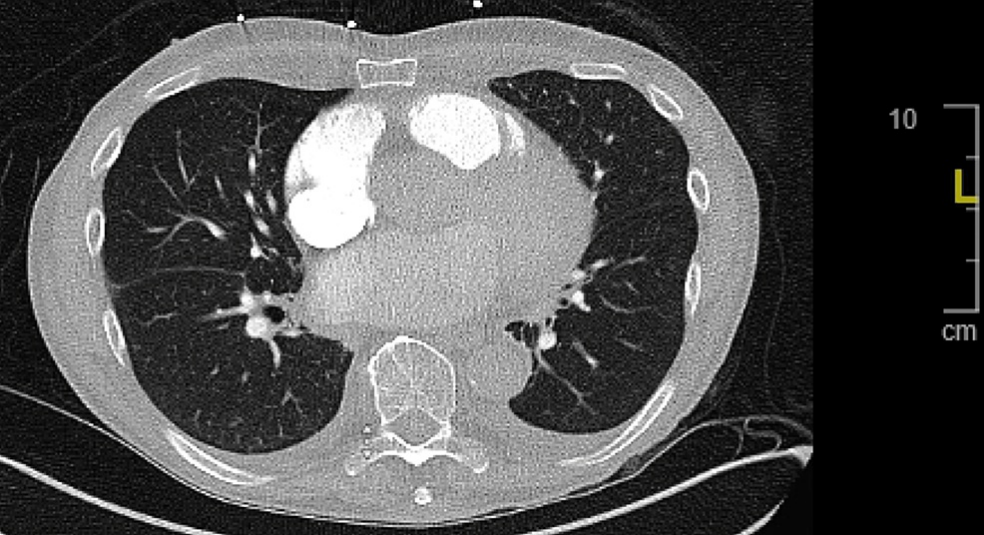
Pulmonary CT axial view showing intralobular septal thickening
